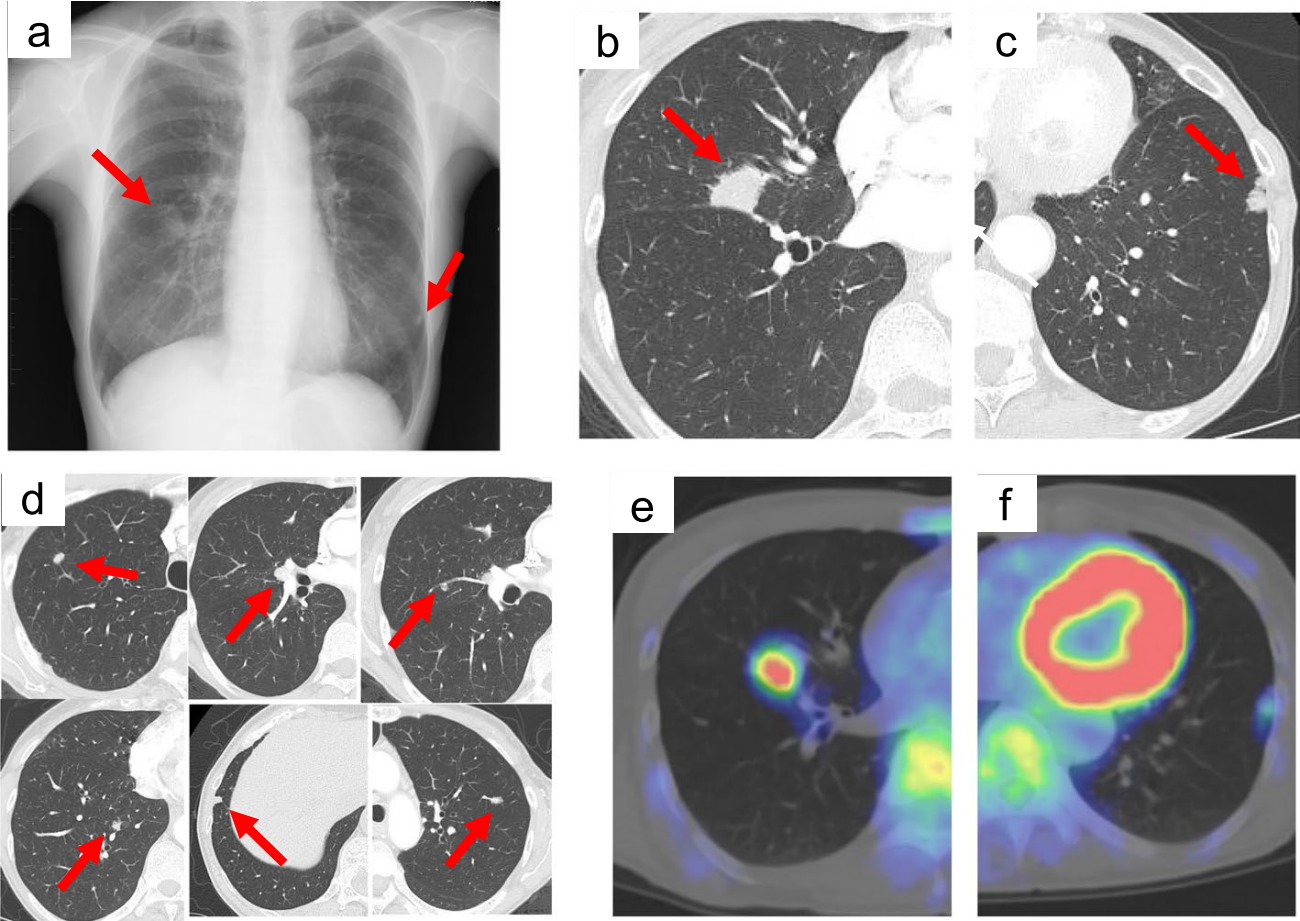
a Chest X-ray findings showing nodular shadows on the right middle and left lower lung field (arrows). b Chest CT showing a 1.8 × 1.6 cm nodule in the right middle lobe (arrow). c A 1.0 × 0.7 cm nodule in the left lower lobe (arrow). d Small nodules in both lungs (arrows). e FDG-PET-CT findings showing an increase in FDG accumulation in the nodule located in the right middle lobe (SUV_max_: 2.9). f An increase in FDG accumulation in the nodule located in the left lower lobe (SUV_max_: 1.1). CT, computed tomography; FDG, fluorodeoxyglucose; PET, positron emission tomography
